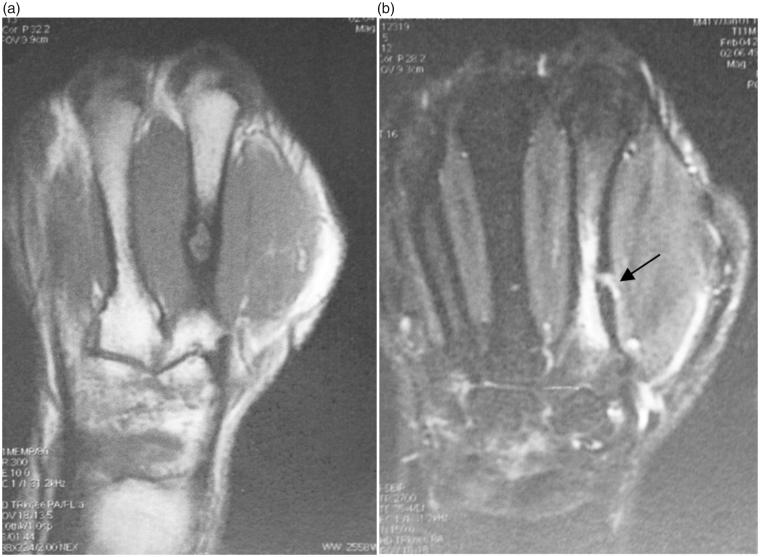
(a,b) MRI of the hand shows an intraosseous second metacarpal geode and the path of the fistula indicated by the black arrow.
